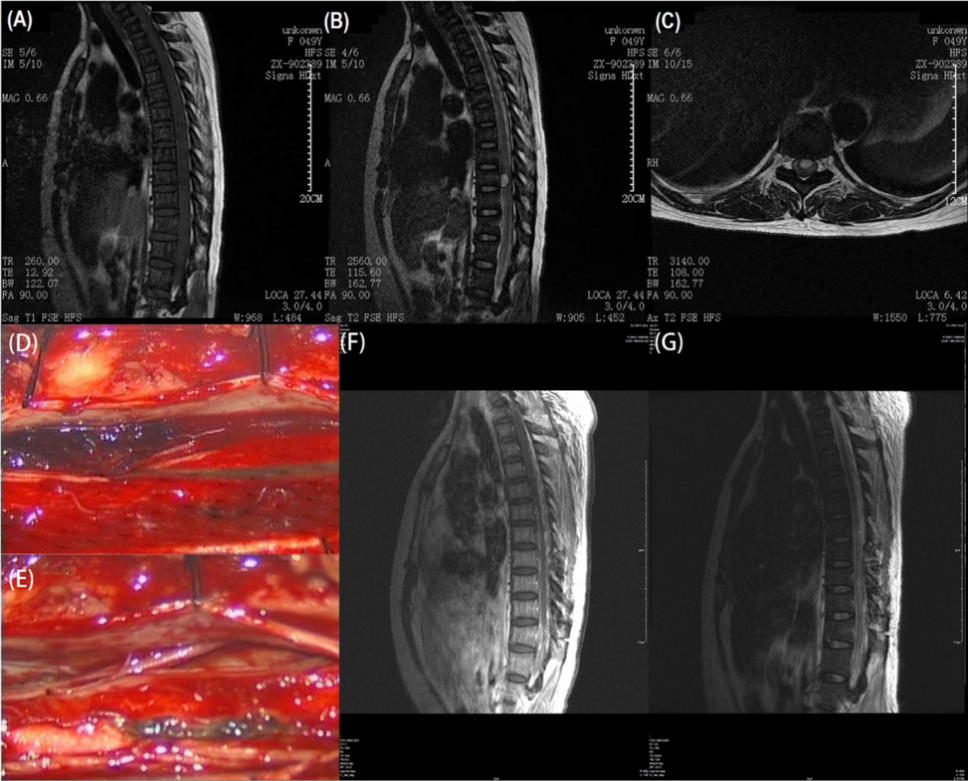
Radiological and intraoperative findings of the presented case of spontaneous acute spinal subdural hematoma. T1-weighted magnetic resonance imaging showed an isointensive lesion situated at the T9 level, ventral to the spinal cord (A). T2-weighted magnetic resonance images showed the lesion was a well-demarcated, oval-shaped extramedullary mass at the T9 level (B, C). An emergency laminectomy from T8 to T10 was performed and only a hematoma was found during the operation (D) and it was successfully evacuated (E). Postoperative sagittal T1-weighted contrast enhanced (F) and T2-weighted (G) magnetic resonance imaging showed total removal of that hematoma.
